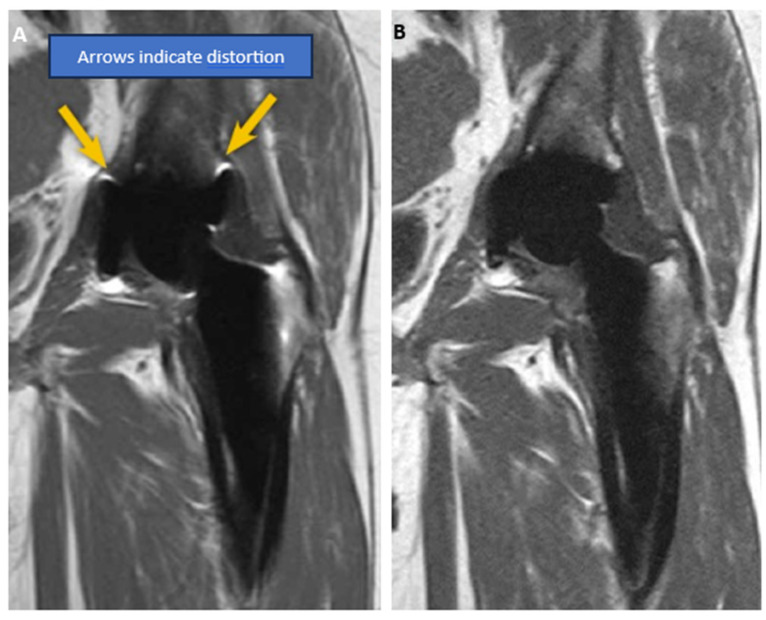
Same patient’s MR images of conventional hip metal implant: (A) metal-induced artifact present in MR image; (B) no distortion present in MR image [204].
